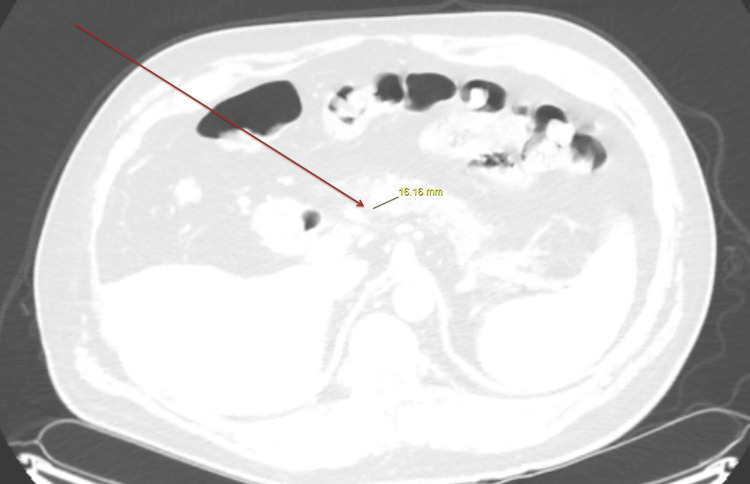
Ga-68 PET-CT with 16-mm hypermetabolic, hyper-vascular lesion in the pancreatic neck-body junction representing pancreatic neuroendocrine tumor
